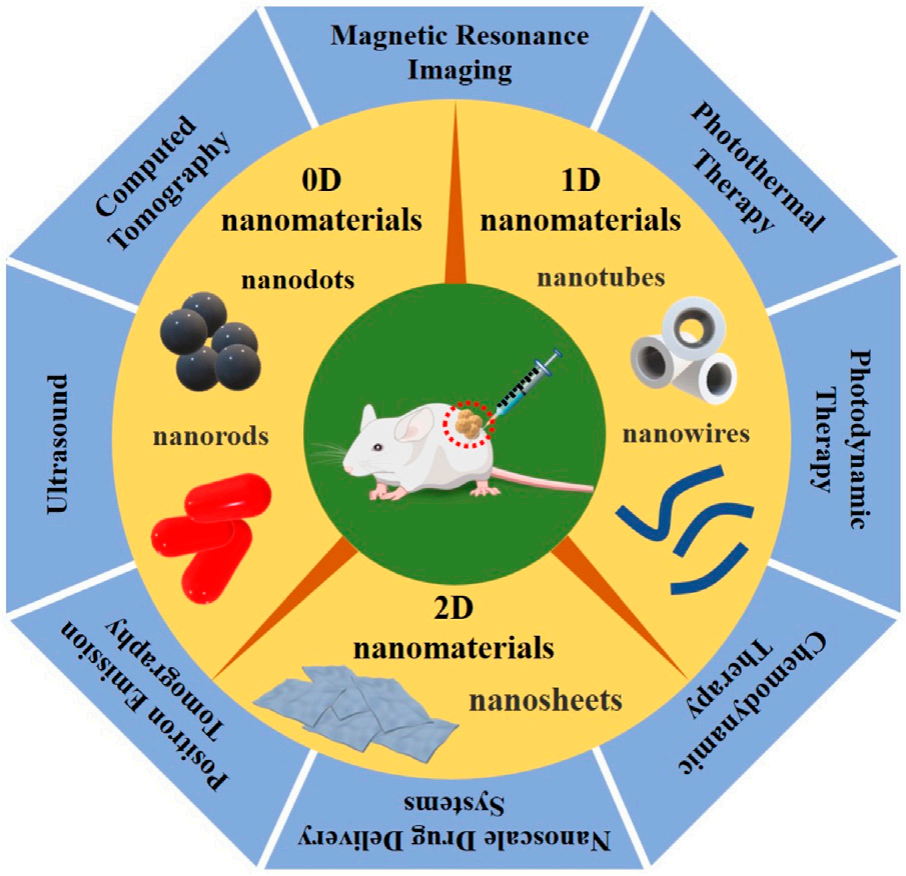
Graphical summarization of the three types of LDNs, according to dimensional and structure characteristics, divided into 0D nanomaterials (such as quantum dots and nanorods), 1D nanomaterials (such as nanowires and nanotubes), and 2D nanomaterials (such as nanosheets), combined with the novel therapeutic methods and imaging techniques, including PTT/PDT/CDT/NDDS and MRI/US/PAI/CT/PET, which have great promise for imaging-guided non-invasive cancer therapy.
